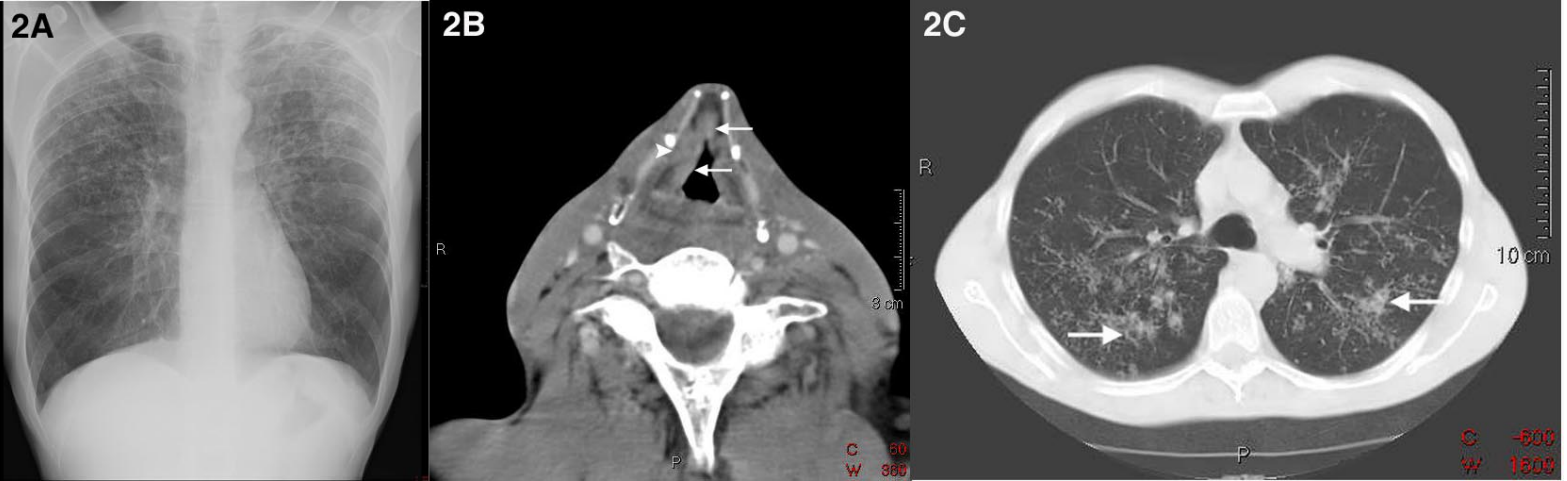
Radiography. (A) Initial antero-posterior chest X-ray. Miliary nodules predominantly bilaterally distributed in the upper parts of the lung parenchyma. (B) Axial contrast-enhanced computed tomography image of the neck: enhanced mass (arrows) in the false vocal cord which extends into the anterior commissure and obliteration of the paraglottic fat (arrowhead). (C) Axial high resolution computed tomography image of the chest: diffusely distributed interstitial nodular alterations with formation of central cavities (arrows).
